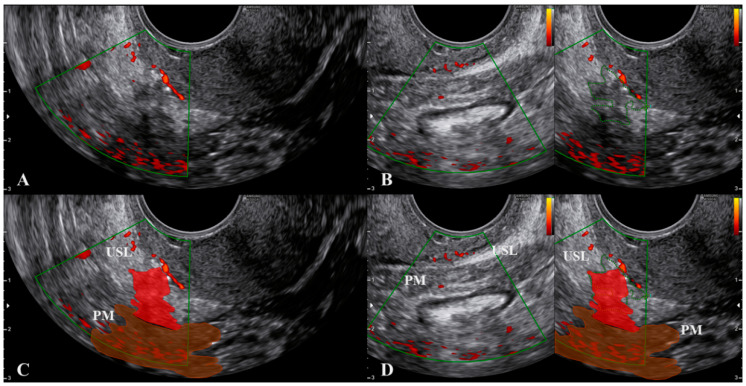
Visualization of the small vessels within the lowest part of the uterosacral ligament using color Doppler. Figure (C,D) are the marked images of figure (A,B), respectively. Endometriosis lesions of the uterosacral ligament and parametrium are in red. Abbreviations: PM, parametrium; USL, uterosacral ligament.
